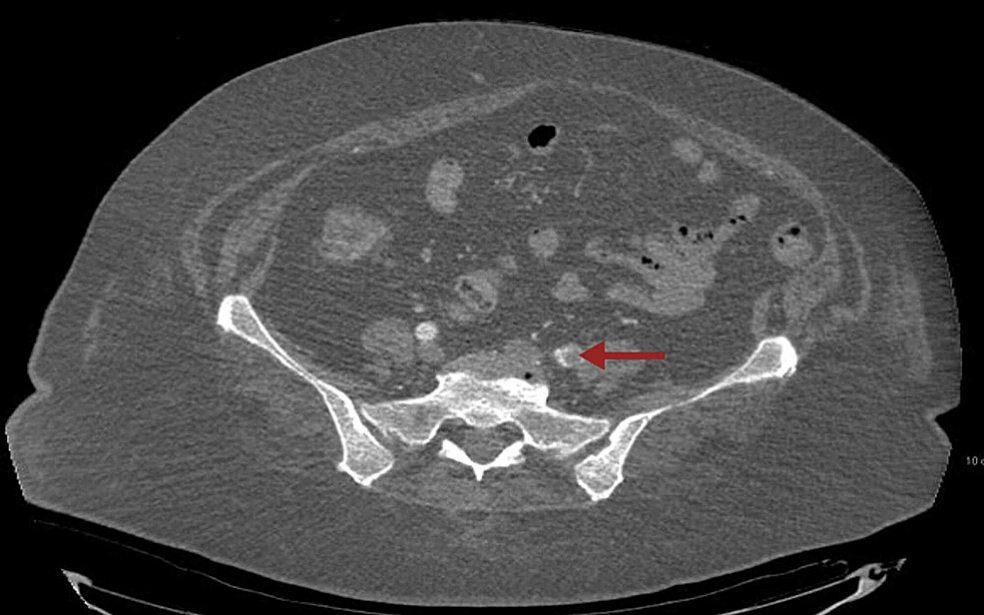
CT angiogram of the abdomen with the arrow pointing towards thrombus in the left common femoral artery.
